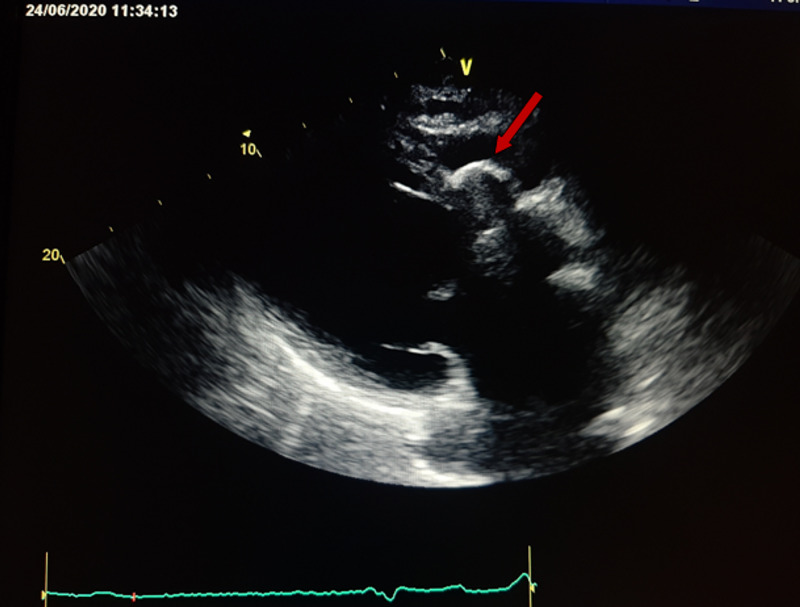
Transthoracic echocardiography showing cyst in the basal part of interventricular septum in the parasternal long-axis view
